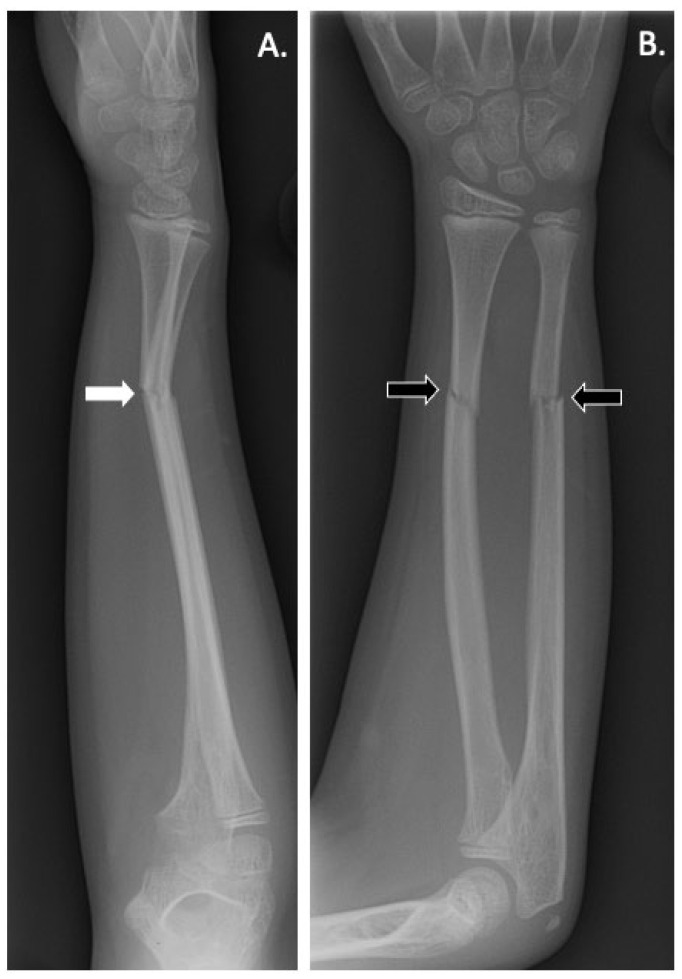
(A) Lateral and (B) anteroposterior radiographs of the right forearm of a patient with *LRP5* variant-induced familial exudative vitreoretinopathy (FEVR) showing radial and ulnar fractures (black arrows). Angulation is noted on the lateral X-ray (white arrow) suggesting possible instability and loss of physiological position.
